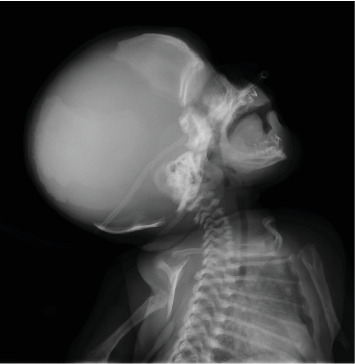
Lateral radiograph of the calvarium. Wide sutures and fontanels.
